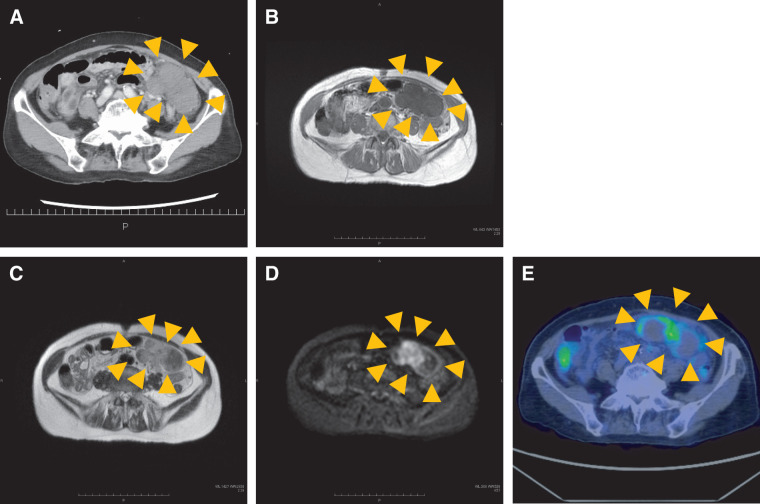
Preoperative radiologic findings. (A) Contrast-enhanced CT at presentation demonstrates an approximately 80-mm, relatively homogeneous mass in the left abdomen with poor internal enhancement. (B) T1-weighted image shows uniformly low signal intensity within the tumor. (C) T2-weighted image demonstrates heterogeneous signal intensity, consisting of intermediate-signal areas and distinct low-signal regions. (D) DWI shows high signal intensity in the intermediate T2-signal areas, whereas the T2-low-signal regions appear hypointense. (E) PET-CT shows SUVmax: 4.6 accumulation along the edge of the tumorous lesion, with no abnormal uptake elsewhere. DWI, diffusion-weighted imaging; SUVmax, maximum standardized uptake value
